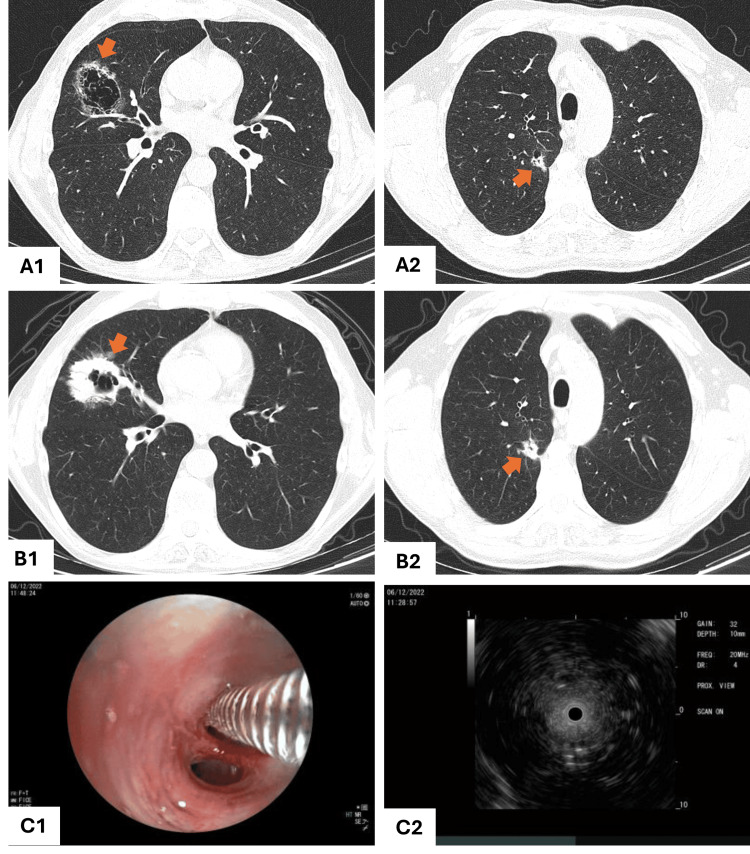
Serial chest CT and bronchoscopic assessment of progressive cystic lung lesions (A1, A2) Baseline axial chest CT showing a dominant cystic lesion in the middle lobe and a smaller cystic lesion in the right upper lobe. At baseline, the dominant lesion measured 42 mm, with a wall thickness of 5 mm and the presence of mural nodularity. (B1, B2) Follow-up CT after 12 months, showing interval enlargement with the dominant lesion measuring 56 mm, increased wall thickness, and development of spiculated margins. (C1) Bronchoscopic view of the segmental bronchus leading to the middle lobe lesion. (C2) Radial EBUS image demonstrating a concentric probe-within-lesion pattern. The miniprobe is centrally located within a circumferential solid lesion, which shows predominantly hypoechoic, heterogeneous echotexture with scattered internal hyperechoic foci and loss of the normal aerated lung artifact. EBUS: Endobronchial ultrasound.
